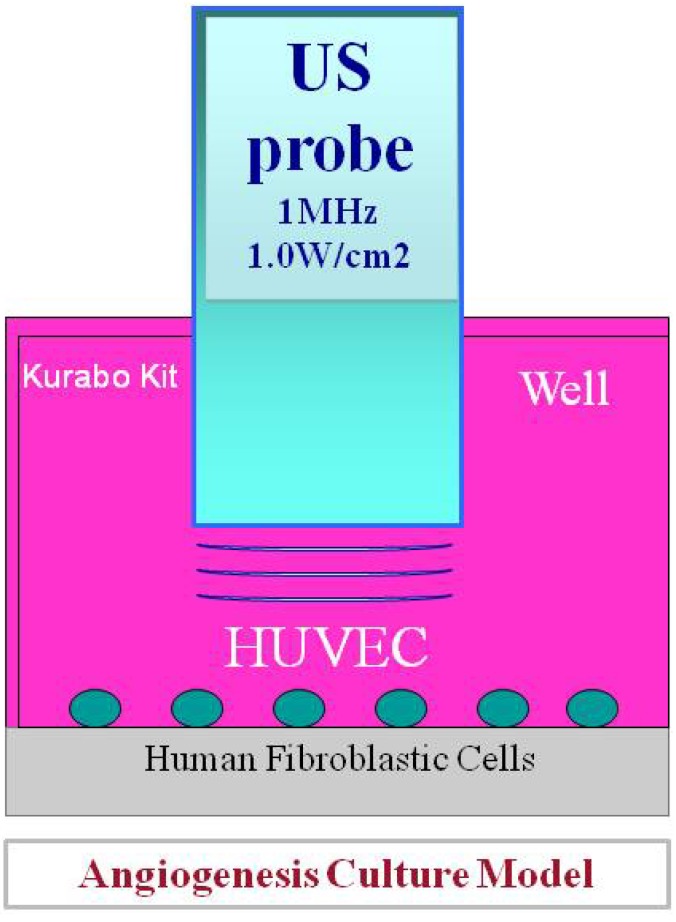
A figure showing a suppression experiment of angiogenesis by ultrasound, designed by the author *et al.* Irradiate ultrasound (using Sonitoron2000: Richmar, USA) per each 1 well under low-output conditions in the state in which human umbilical vascular endothelial cells (HUVEC) and human skin fibroblasts were co-cultivated (KURABO, Japan).
